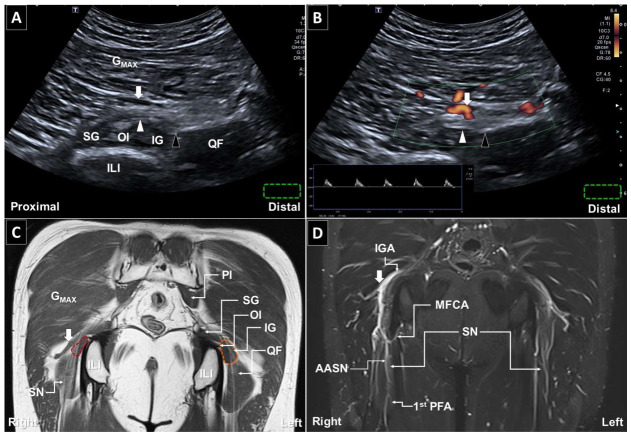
Long−axis ultrasonographic B−mode (A) and Doppler−mode (B) images demonstrate sciatic nerve entrapment by the inferior gluteal artery (white arrow). The site of entrapment (white arrowhead) and focal swelling of the sciatic nerve (black arrowhead) are seen. Corresponding coronal T1−weighted (C) and contrast-enhanced T1−weighted (D) magnetic resonance images demonstrate engorgement of the inferior gluteal artery on the right side (white arrow) compared with the contralateral side (orange dashed line), accompanied by atrophy of the inferior gemellus muscle (red dashed line). IGA, inferior gluteal artery; SN, sciatic nerve; AASN, accompanying artery of the sciatic nerve; PI, piriformis muscle; GMAX, gluteus maximus; SG, superior gemellus; IG, inferior gemellus; OI, obturator internus; QF, quadratus femoris; ILI, ilium; MFCA, medial circumflex femoral artery; 1st PFA, first perforating artery. The ultrasonographic images were obtained from a 50−year-old man who presented with persistent tightness and pain in the right gluteal region and posterior thigh for more than two years. According to his description, the symptoms had been noticeable since adolescence; however, the pain progressively worsened over the past two years with prolonged sitting at work and weight gain. Following the initiation of gym-based gluteal strengthening and bicycle training, the symptoms became more severe. The patient had no relevant comorbidities, including thyroid disease or diabetes mellitus. Previous conservative treatments, including lumbar traction and ultrasound-guided injections targeting the lumbar facet joints, piriformis muscle, and caudal epidural space, provided only limited symptomatic relief.
